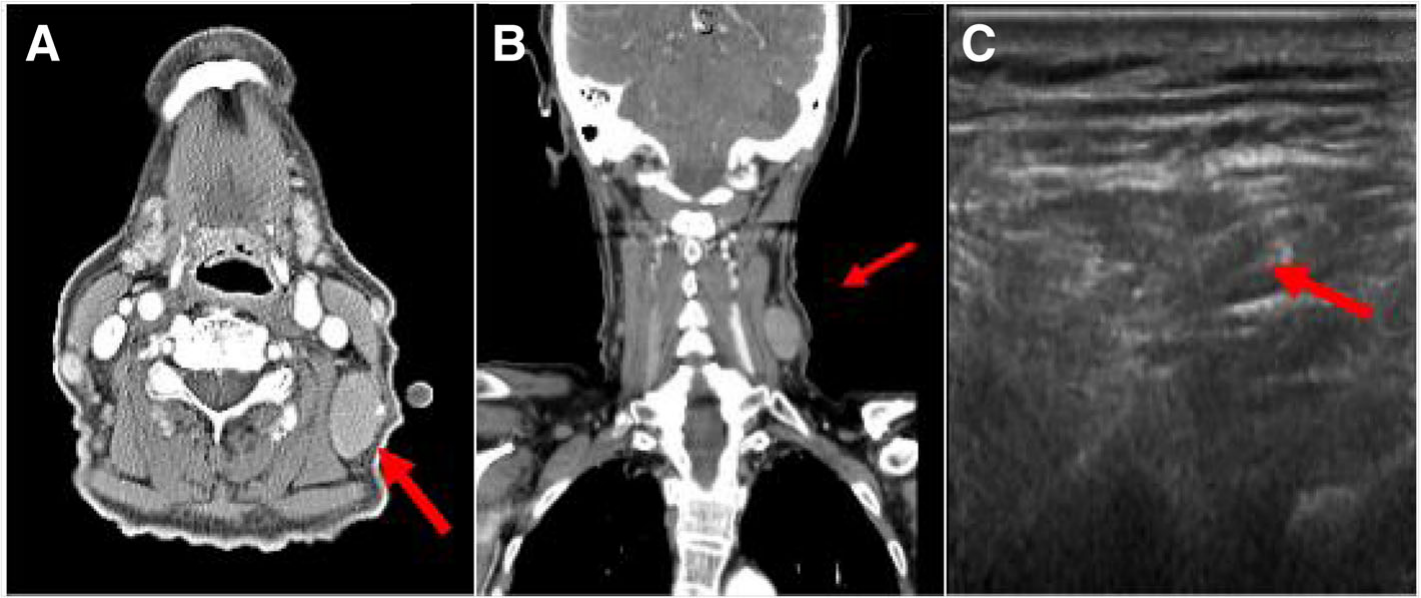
Initial neck computed tomography for cervical mass and follow-up neck ultrasound. a and b Neck computed tomography showing 4.5 cm level III left-sided neck mass (red arrows). c Neck ultrasound showing resolution of previously observed neck mass. Small non-pathologic appearing nodes with fatty hila were identified (red arrow)
